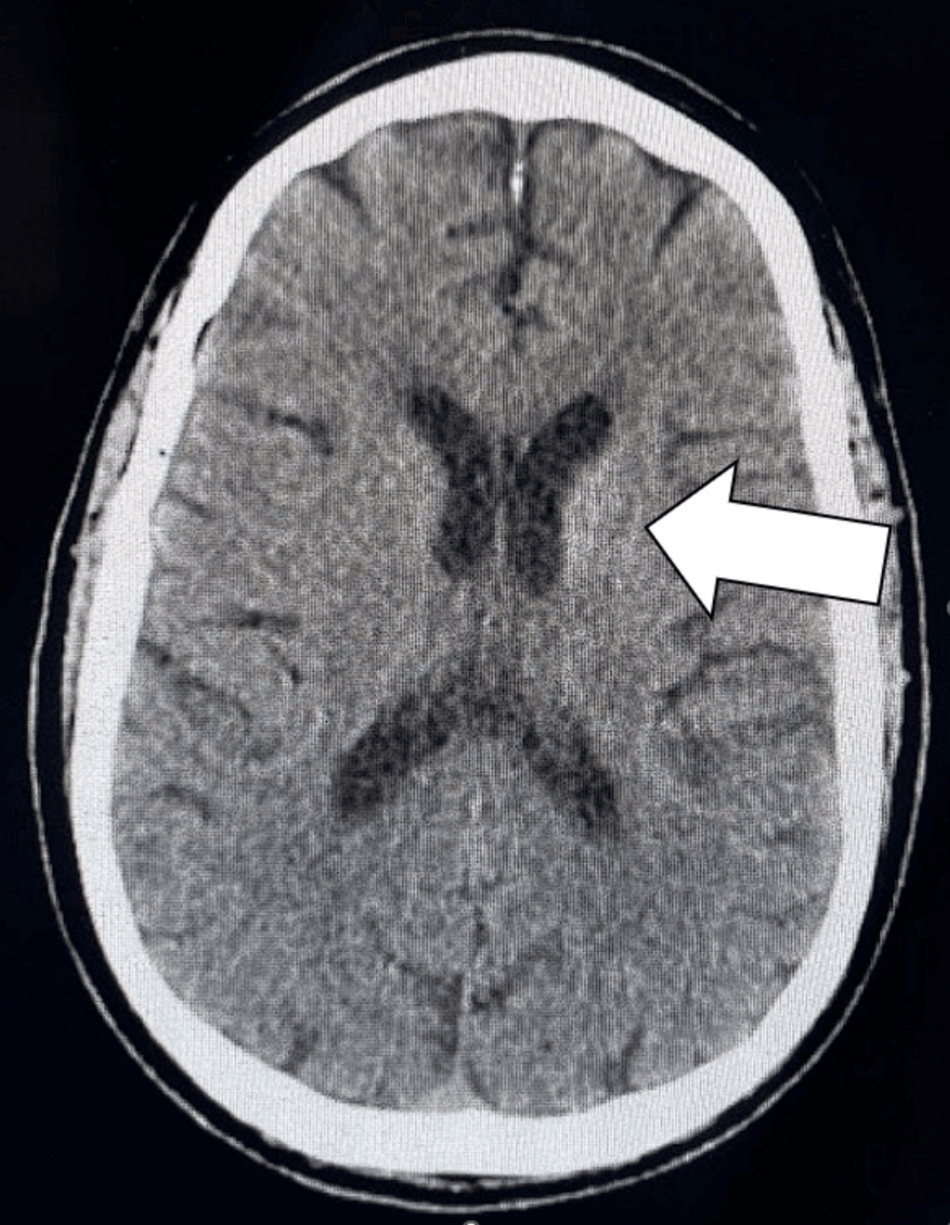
CT Scan of the Brain White Arrow: Prominent density on the left side of the patient's brain
